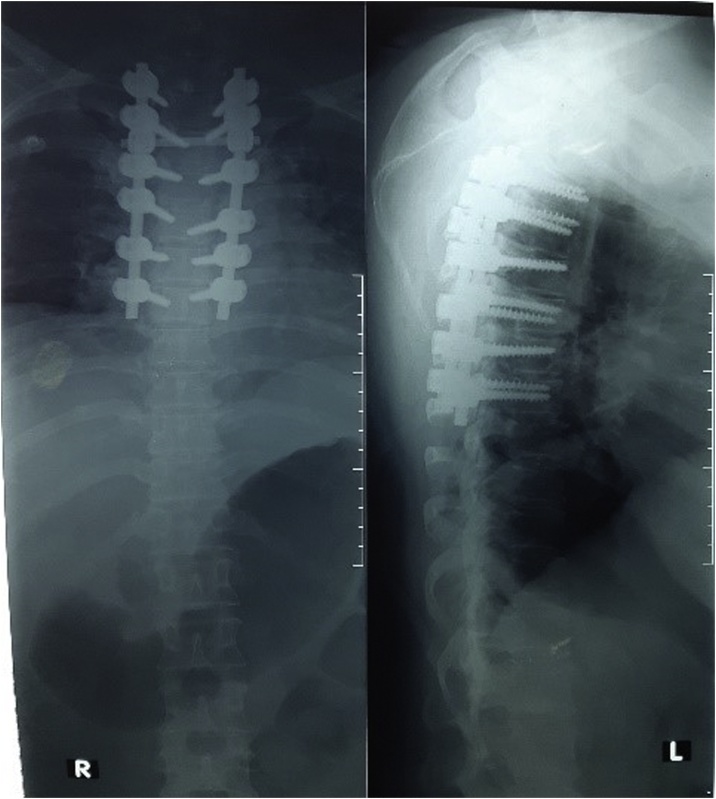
The postoperative radiograph showed pedicle screw and rod placement for posterior stabilization.
